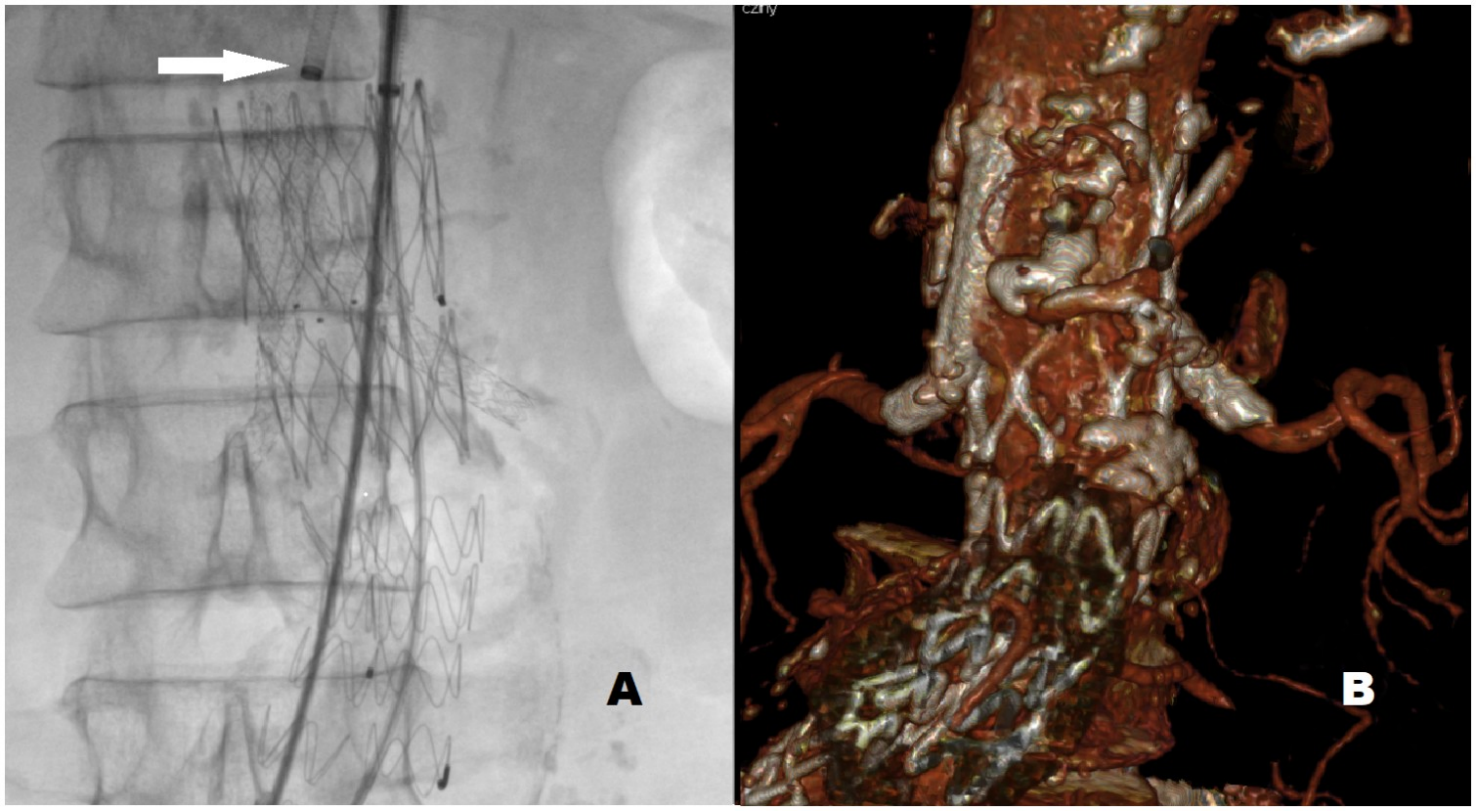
Stentgraft and chimney stents after implantation. A. Angiographic picture during the procedure (arrow -guide catheter for chimney stent implantation and pressure wire delivery). B. Follow up computed tomography after 6 months—patency of both chimney stents.
